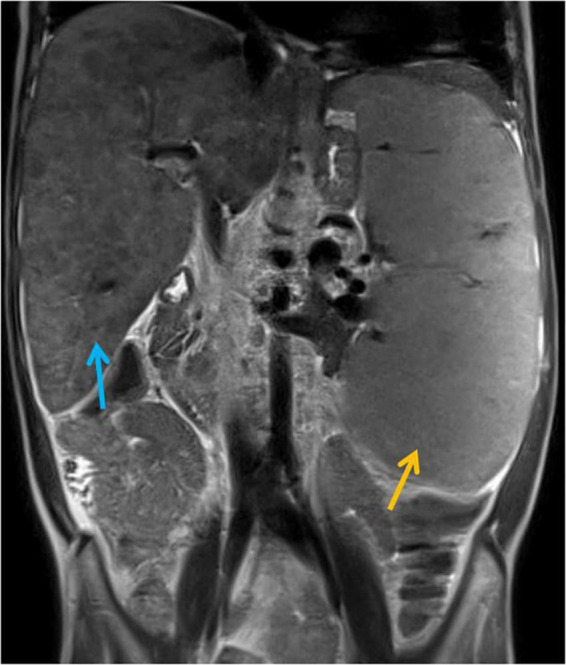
Magnetic Resonance Imaging (MRI) examination of the abdominal cavity, T2-weighted coronal image. Massive hepatosplenomegaly (the liver and spleen are marked with blue and orange arrows, respectively) and granulomatous lesions in the liver and spleen resulting in severe portal hypertension, hypersplenism, and ultimately, hepato-renal syndrome.
